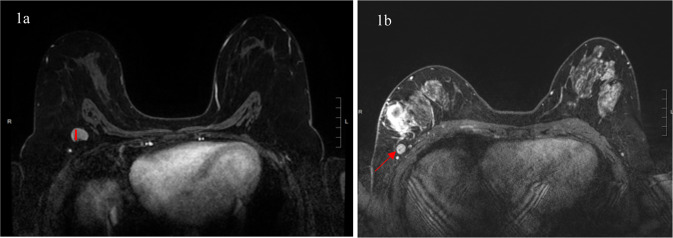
Abnormal lymph node findings on breast MRI. a, b Examples of lymph node abnormalities on breast MRI. a Left: shows increased cortical thickening (≥3 mm) of an axillary lymph node in the right axilla (red line). b Right: shows an example of an axillary lymph node with rounded morphology in the right axilla and asymmetry when compared to the contralateral axilla (red arrow).
